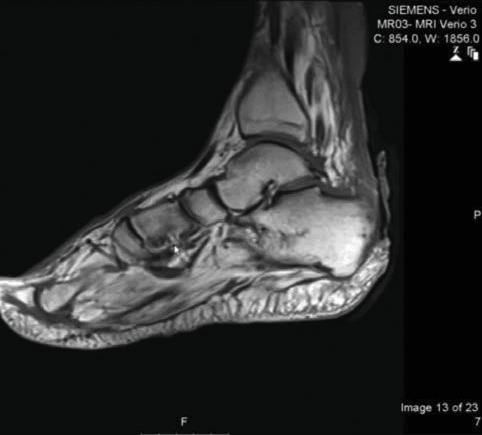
Lateral MRI view of left foot.
